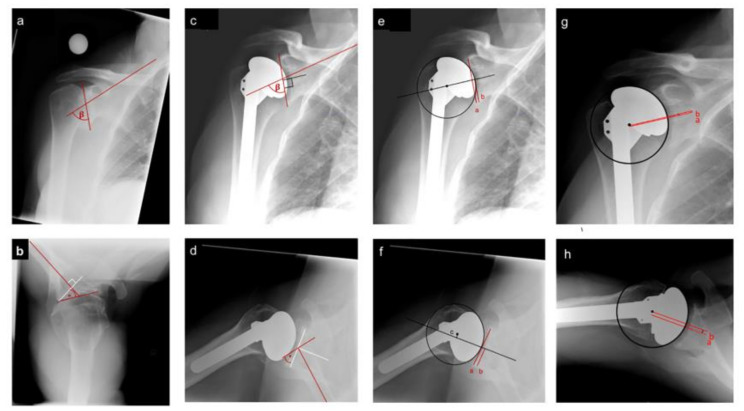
Radiographic measurements: (a) Pre-operative inclination = 90−β, where β is the angle between the supraspinatus fossa and a line from the superior to inferior glenoid. (b) Pre-operative version = the angle (*) between a line connecting the anterior to posterior glenoid and a perpendicular line to the mid-scapular blade. (c) Glenoid component inclination = 90−β. Angle β is the angle between the supraspinatus fossa and a perpendicular line to the mid-component axis. (d) Glenoid component version = the angle (*) between a line perpendicular to the mid-component axis and a perpendicular line to the mid-scapular blade. (e) Glenoid component offset SI = difference between the midpoint of a line connecting the superior to inferior glenoid and length of a line connecting the midpoint of the glenoid component to the inferior glenoid. (f) Glenoid component offset AP = difference between the midpoint of a line connecting the anterior to posterior glenoid and length of a line connecting the midpoint of the glenoid component to the posterior glenoid. (g) Humeral component offset SI = the difference between line A (a line through the center of the glenoid component) and line B (a parallel line to line A, from the center of the humeral component). (h) Humeral component offset AP = the difference between line A (a line through the center of the glenoid component) and line B (a parallel line to line A, from the center of the humeral component).
